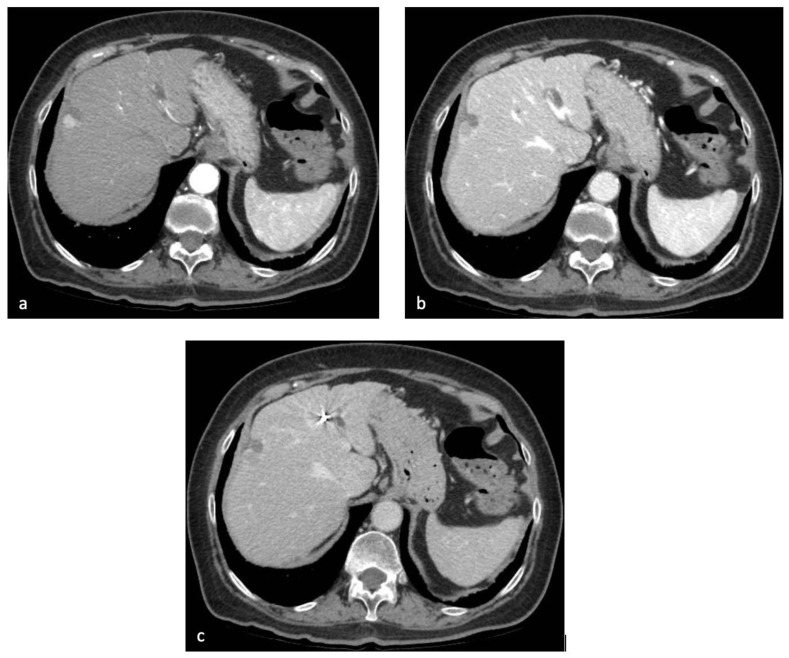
Contrast-enhanced CT of the upper abdomen in the patient discussed in Figure 1. After administration of iodinated contrast agent, the subcapsular lesion showed arterial hyperenhancement (a), with progressive wash-out in the portal-venous (b) and delayed (c) phase.
